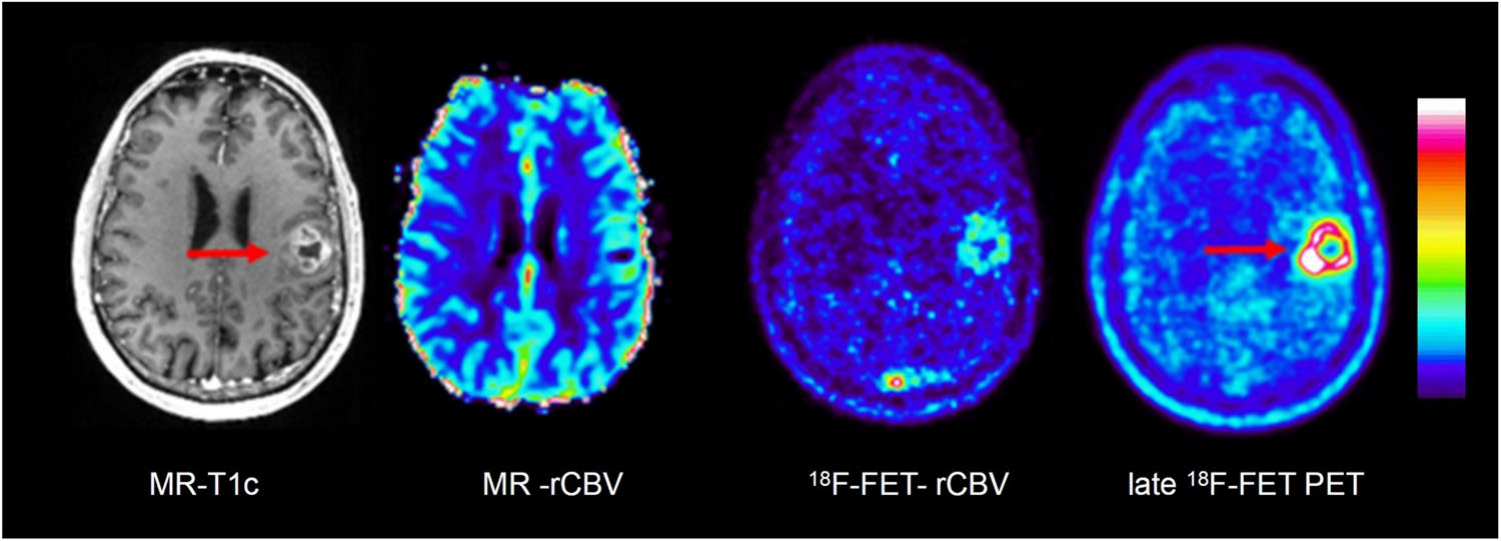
Contrast-enhanced T1-weighted MRI (MR-T1c), MR-rCBV, ^18^F-FET-rCBV and late ^18^F-FET PET (from left to right) in a patient with a newly diagnosed glioblastoma. There is a contrast-enhancing lesion in the left frontoparietal cortex showing a pronounced signal on late ^18^F-FET PET (red arrows) but only a discrete signal in MR-rCBV and ^18^F-FET-rCBV. The findings of MR-rCBV and ^18^F-FET-rCBV are very similar
